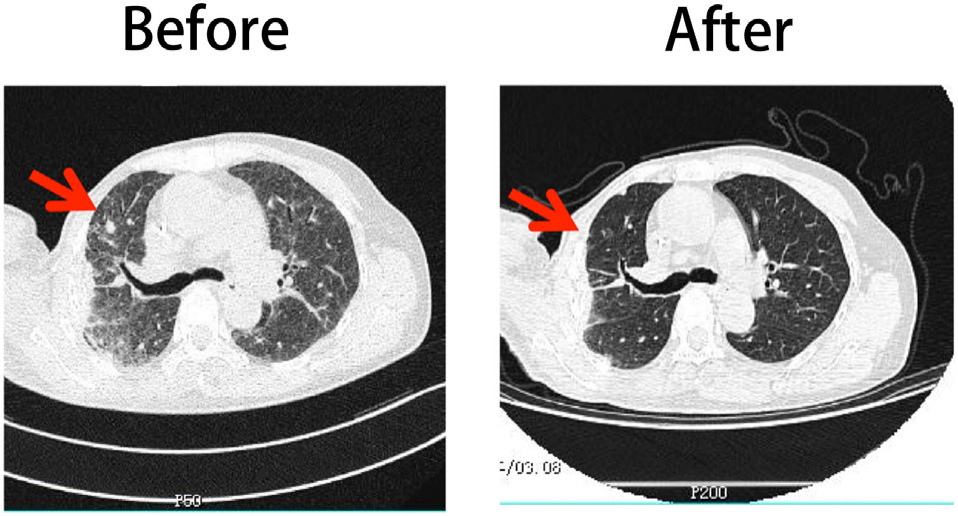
Comparison of the patient’s CT scan images before and after Herceptin-treated natural killer (NK) cell therapy. The left column shows the image obtained before treatment and the right column shows the image obtained after four cycles of NK cell therapy.
